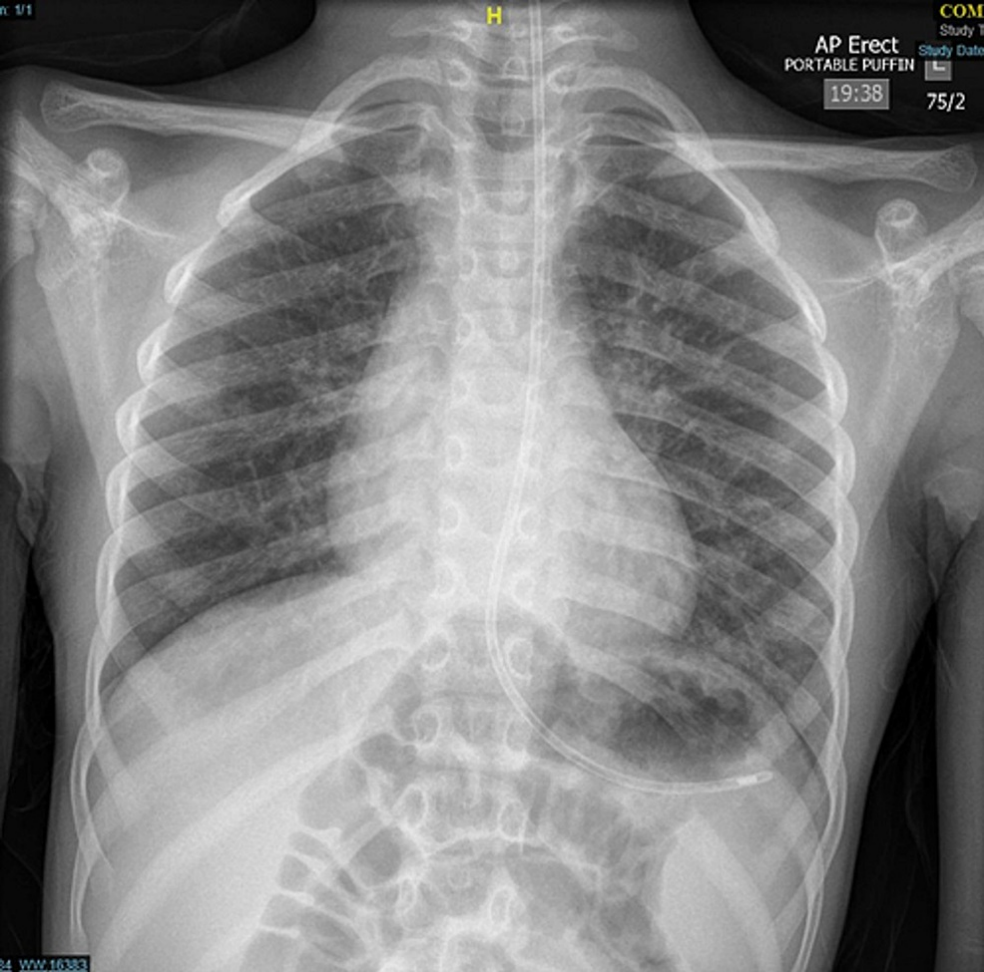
Postoperative chest X-ray Postoperative chest X-ray showing midline trachea and resolution of the widened mediastinum, with a nasogastric tube in situ.
